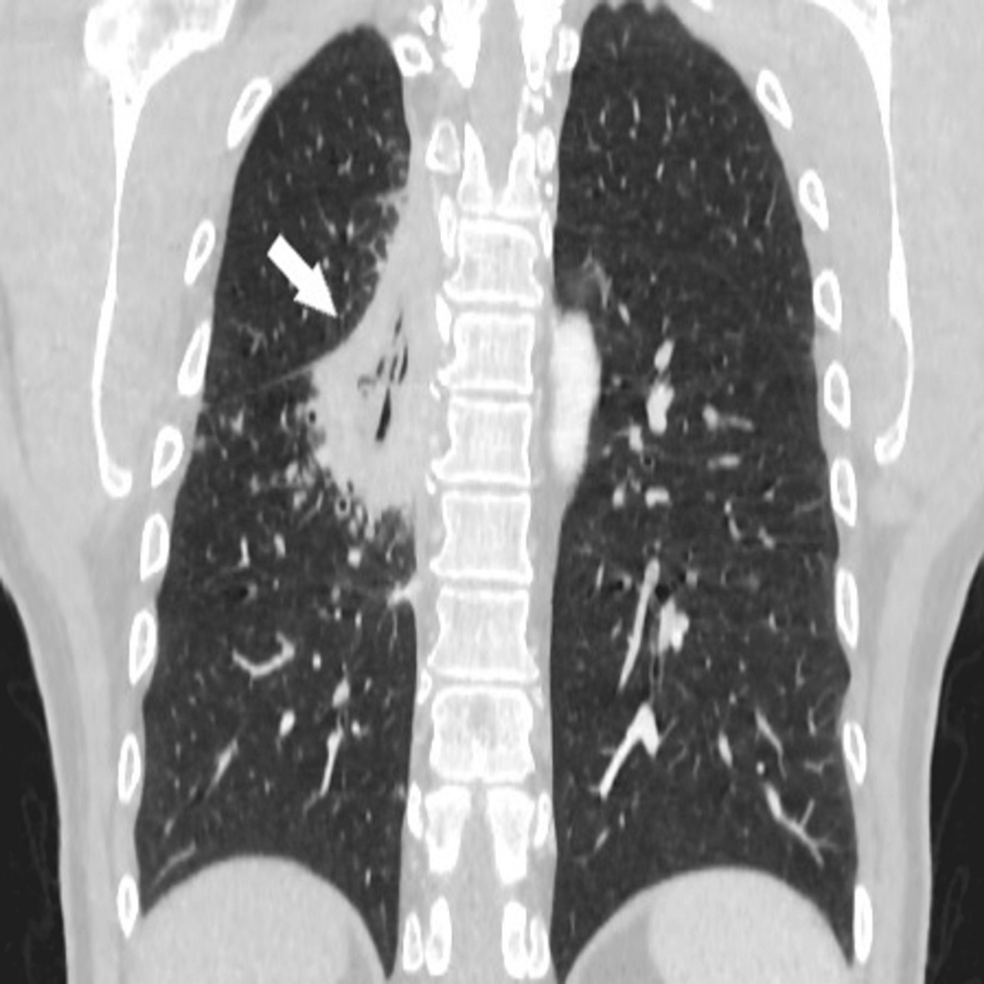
Computed tomography. Coronal images of lung abscess (white arrows).
